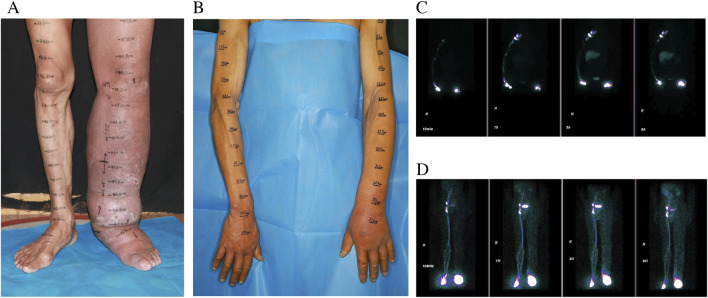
Preoperative pictures of LLL (A) LUL (B), and preoperative lymphoscintigraphy images of LLL [(C) T6] and LUL [(D) T6].
